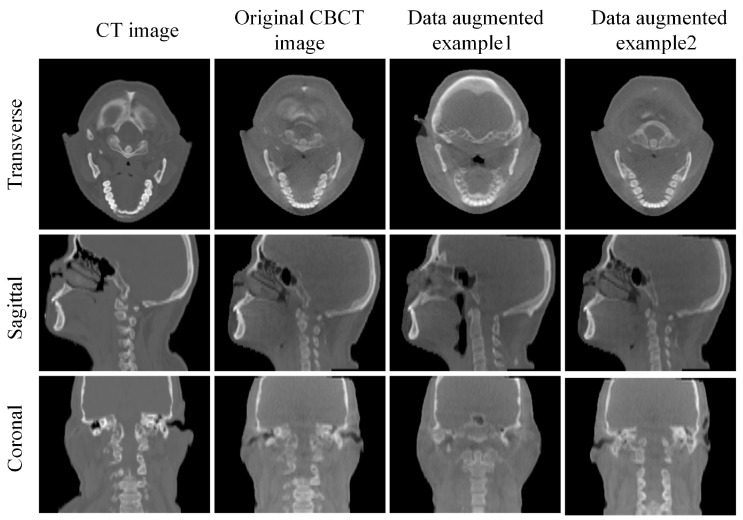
The visualization of the CT image, the original CBCT image, and examples of the original CBCT image after undergoing various rotational and translational data augmentations.
